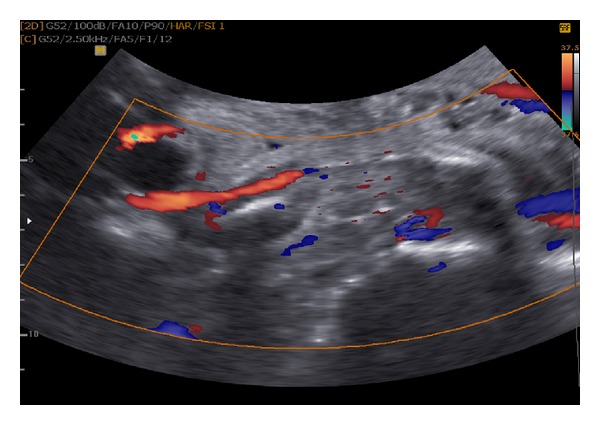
Color Doppler imaging (sagittal view) demonstrated prominent vessels surrounding multiple cystic structure.
